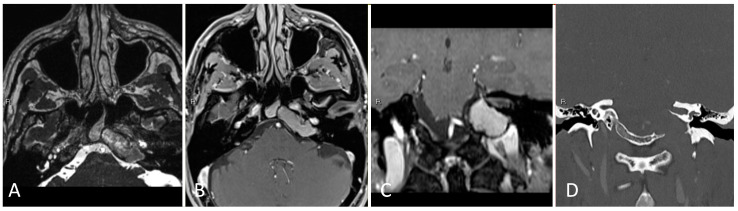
Preoperative imaging of a 43-year-old male with a left petroclival cholesterol granuloma. (A) Axial T2 high-resolution constructive interference steady state (CISS) T2-weighted MRI. (B) Axial T1 post-contrast MRI. (C) Coronal T1 post-contrast MRI. (D) Coronal CTA with contrast.
